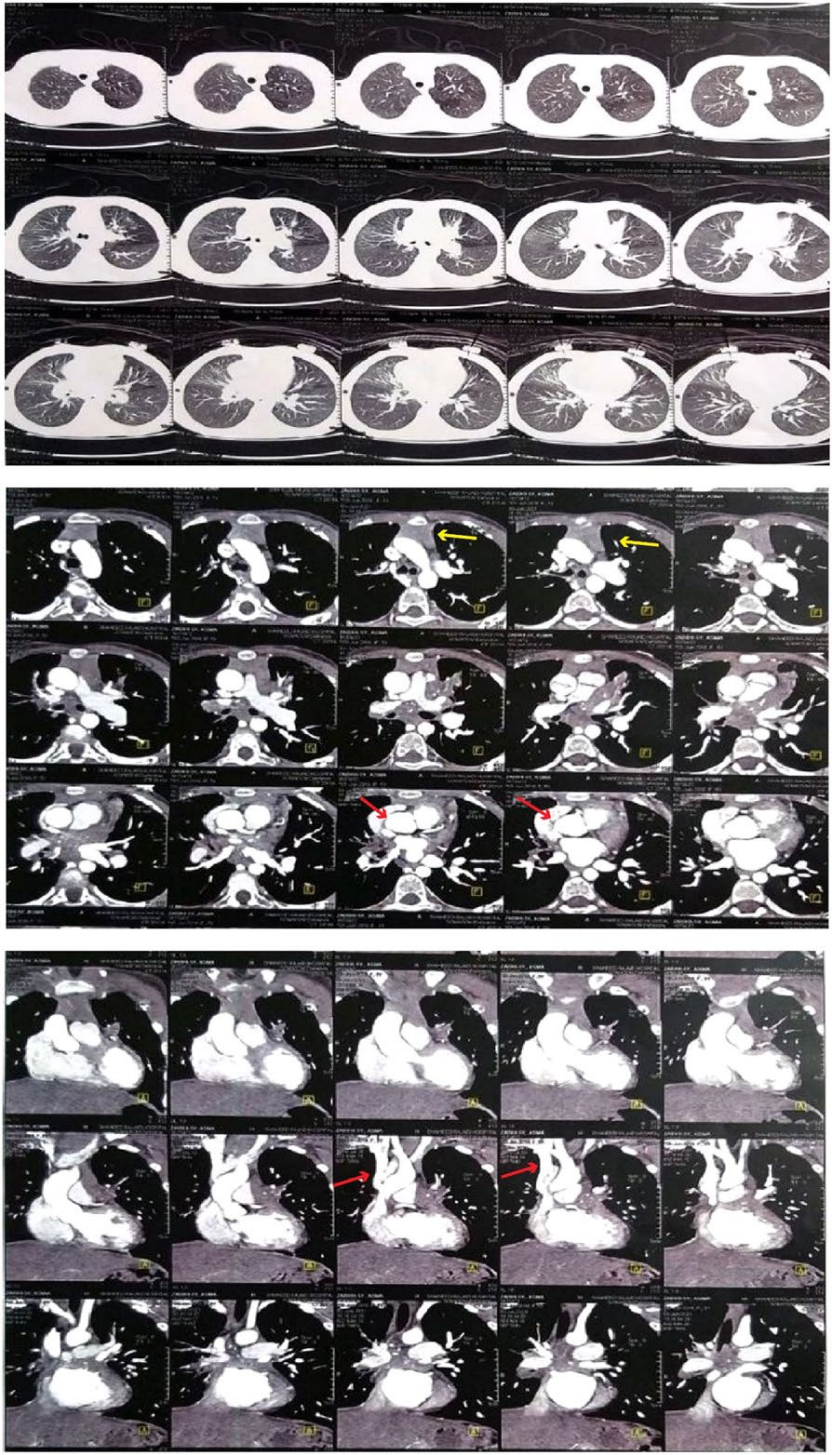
The chest CT angiography of the index patient. Narrowing in the distal part of superior vena cava (red arrows) and mediastinal fat infiltration (yellow arrows) with multiple calcifications
